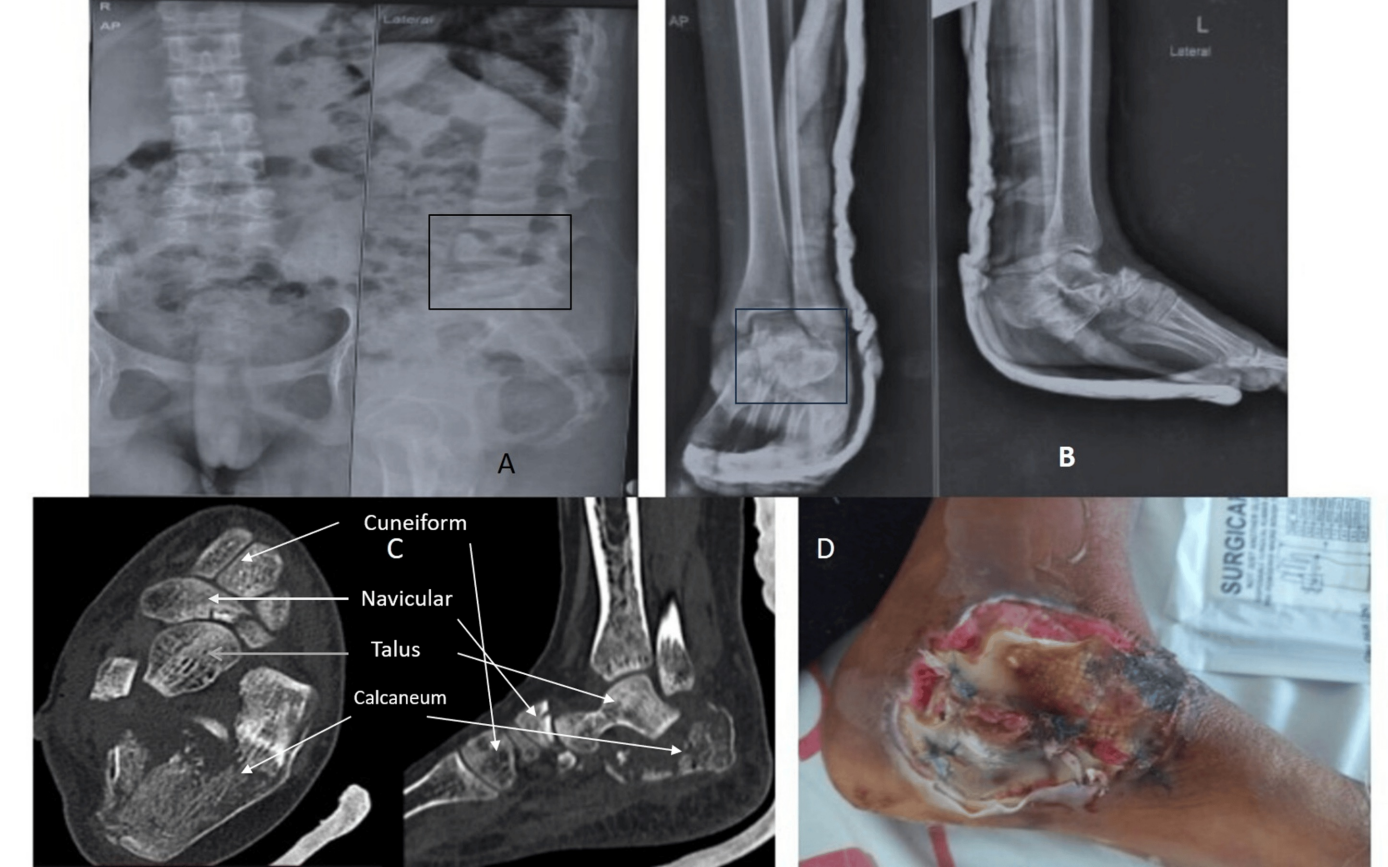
Preoperative scans and wound status. (a) Anteroposterior and lateral radiograph of the lumbar spine showing L4 and L5 wedge compression fracture. (B) Anteroposterior and lateral radiograph of the ankle with foot showing subtalar fracture-dislocation. (C) Cut section of foot CT depicting the pattern of the fracture. (D) Circular wound on the medial aspect of the foot, exhibiting slough and necrotic tissue.
